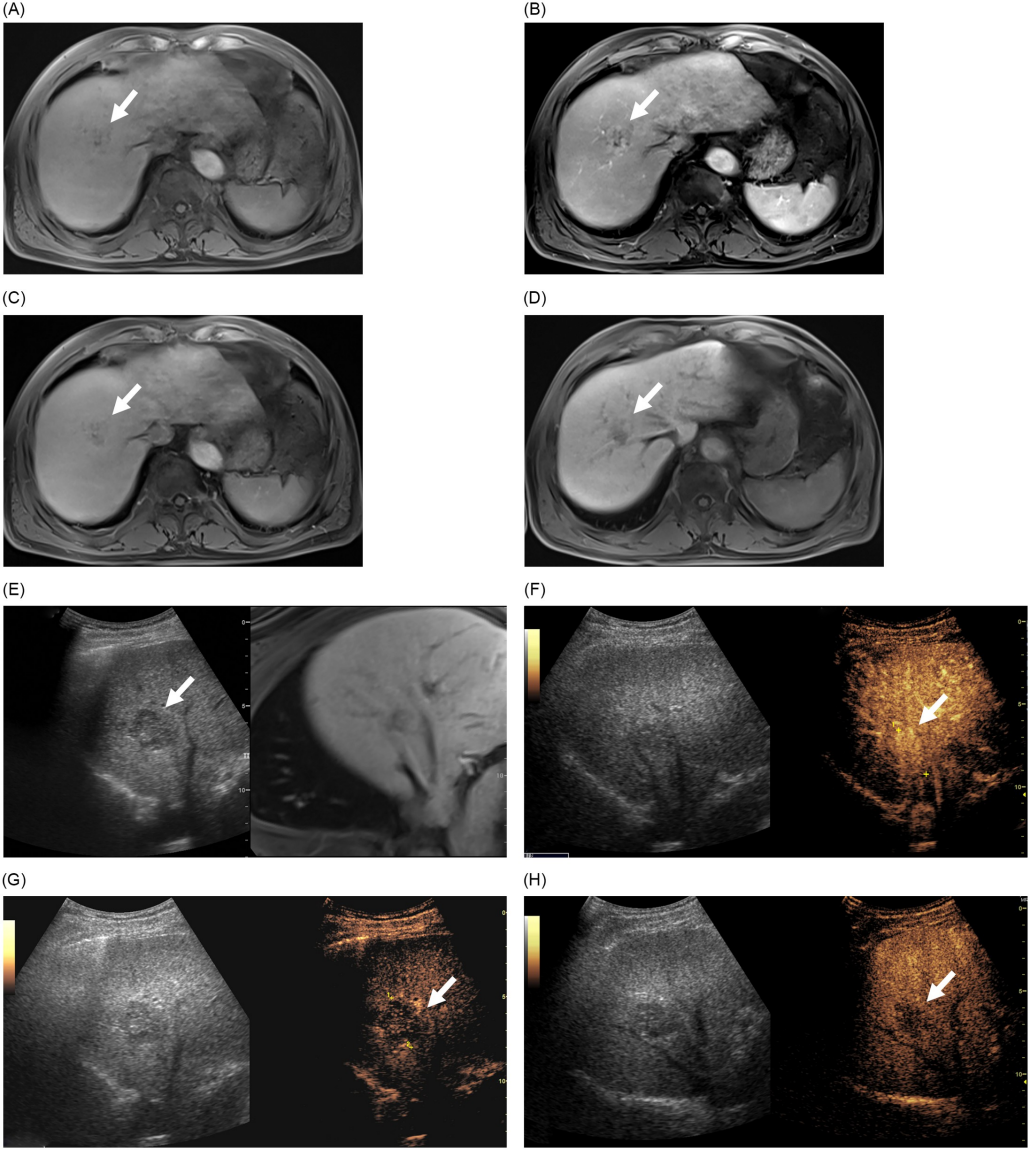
Images in a 72-year-old man with an indeterminate focal liver lesion (arrows) on MRI. An unenhanced MR image shows an irregularly shaped low-to-iso signal intensity lesion in liver segment 8 (A). The lesion shows subtle hyperenhancement on the arterial-phase (B), slightly low signal intensity on the transitional-phase (C), and low-to-iso signal intensity on the hepatobiliary-phase (D). On MRI, the lesion was categorized as indeterminate. Gray scale ultrasound image shows a relatively well-marginated low-echoic mass (E). The lesion shows arterial-phase hyperenhancement (F), late-onset washout (G), and defect on the Kupffer phase (H). On CEUS, it was categorized as probably malignant. The mass was surgically resected and confirmed as a hepatocellular carcinoma.
